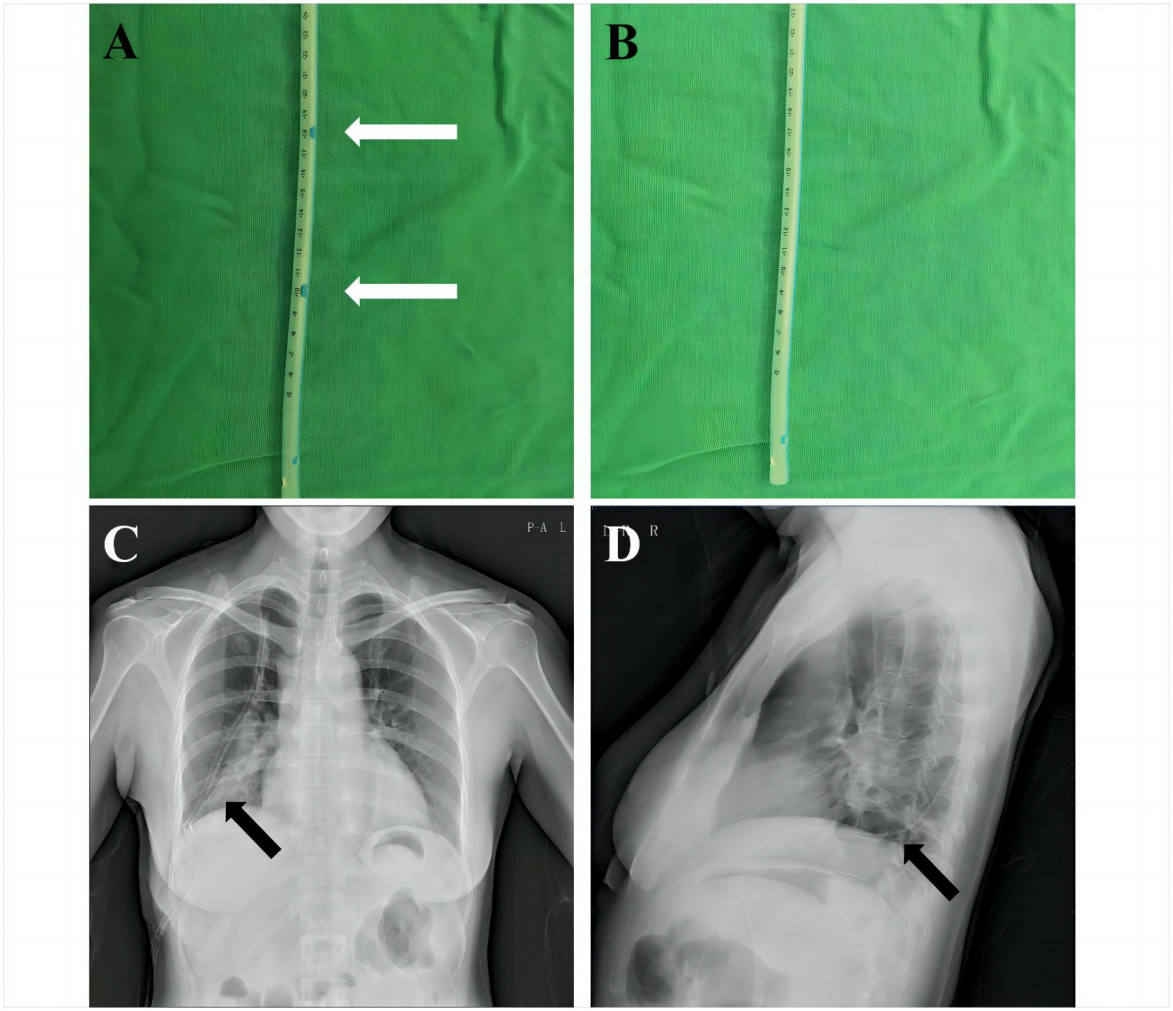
Chest tube management. (A) In the MDG, lateral holes were created at the 5 cm and 18 cm marks of the drainage tube using tissue scissors. (B) In the CDG, the chest tube was not trimmed. As shown in chest X-ray, (C) the tube was inserted from the 7^th^ intercostal space anterior to the midaxillary line, (D) advanced from the posterior mediastinum to the top of the chest cavity, with a depth of 25 cm.
